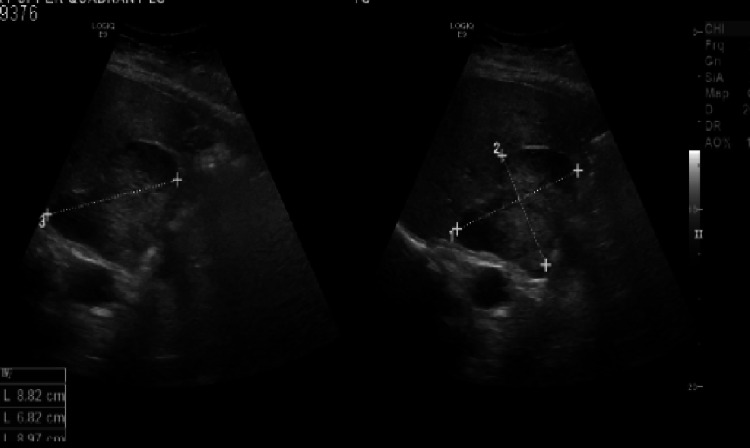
Ultrasonography showing a right adrenal mass
